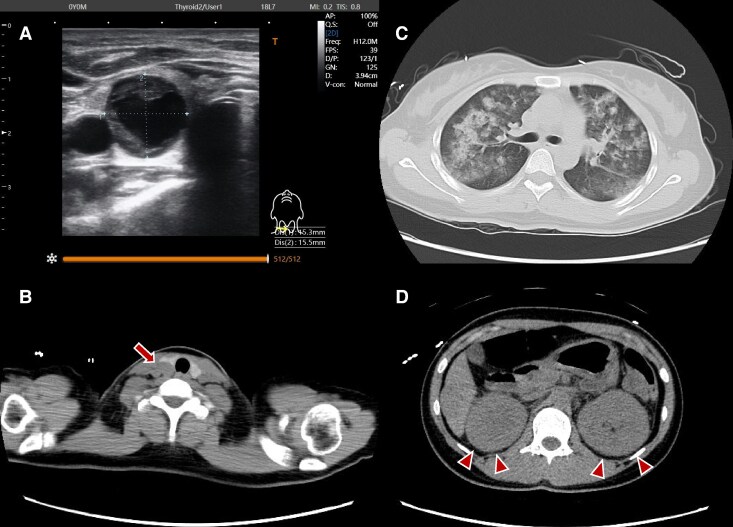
Imaging of the neck, thorax, and abdomen. A, Cervical ultrasonography showing a nodule near the lower pole of the right thyroid lobe. B, Cervical computed tomography (CT) imaging showing a 20-mm mass in the same region (arrow). C, Chest CT showing alveolar opacities, predominantly in the upper lung fields bilaterally. D, Abdominal CT images consistent with acute kidney injury, characterized with bilateral renal enlargement (arrowhead).
